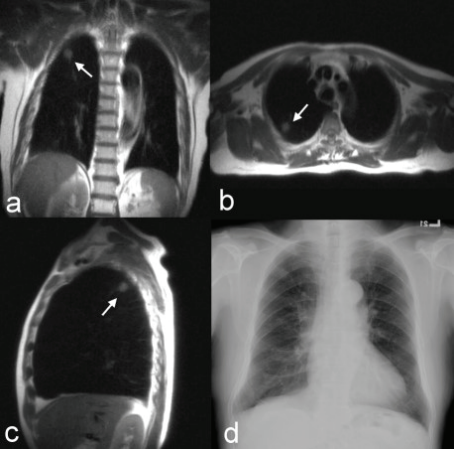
The HASTE images from MRI. The (a) coronal, (b) axial, and (c) sagittal images showed an irregular consolidated mass about 1.2 cm in diameter at the posterior segment of the right upper lobe. Under black blood preparation, the lesion easily stood out from the clear background without the appearance of any vessels. (d) The findings from chest radiography were negative. The nodule was surgically proved to be squamous cell carcinoma, stage Ia.
